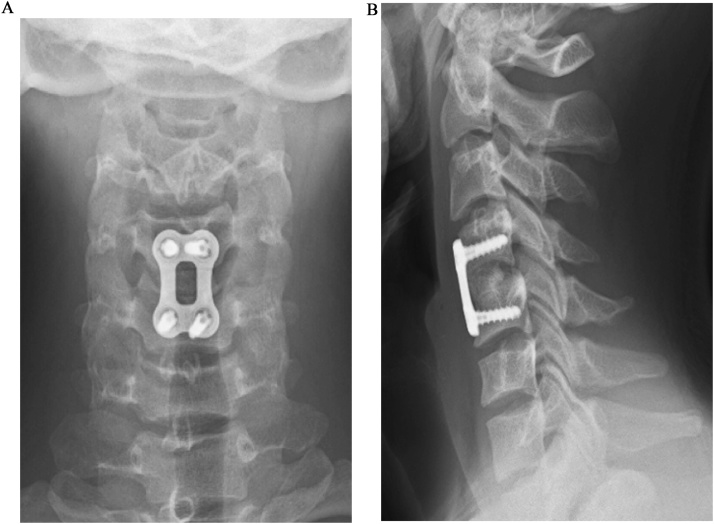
Anteroposterior (a) and lateral (b) radiographs 3 months after ACDF showing complete bony union and 4°-lordosis.
